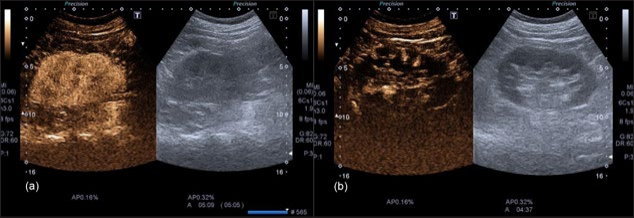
Contrast enhanced ultrasound of the kidneys. 3a: Normal vascular supply of the right kidney with homogeneous enhancement of the cortex. 3b: Minimal cortical enhancement of the left renal cortex, extending from the periphery to the hilum, representing perforating branches of the capsular artery, also known as rim sign, indicative of renal infarction.
